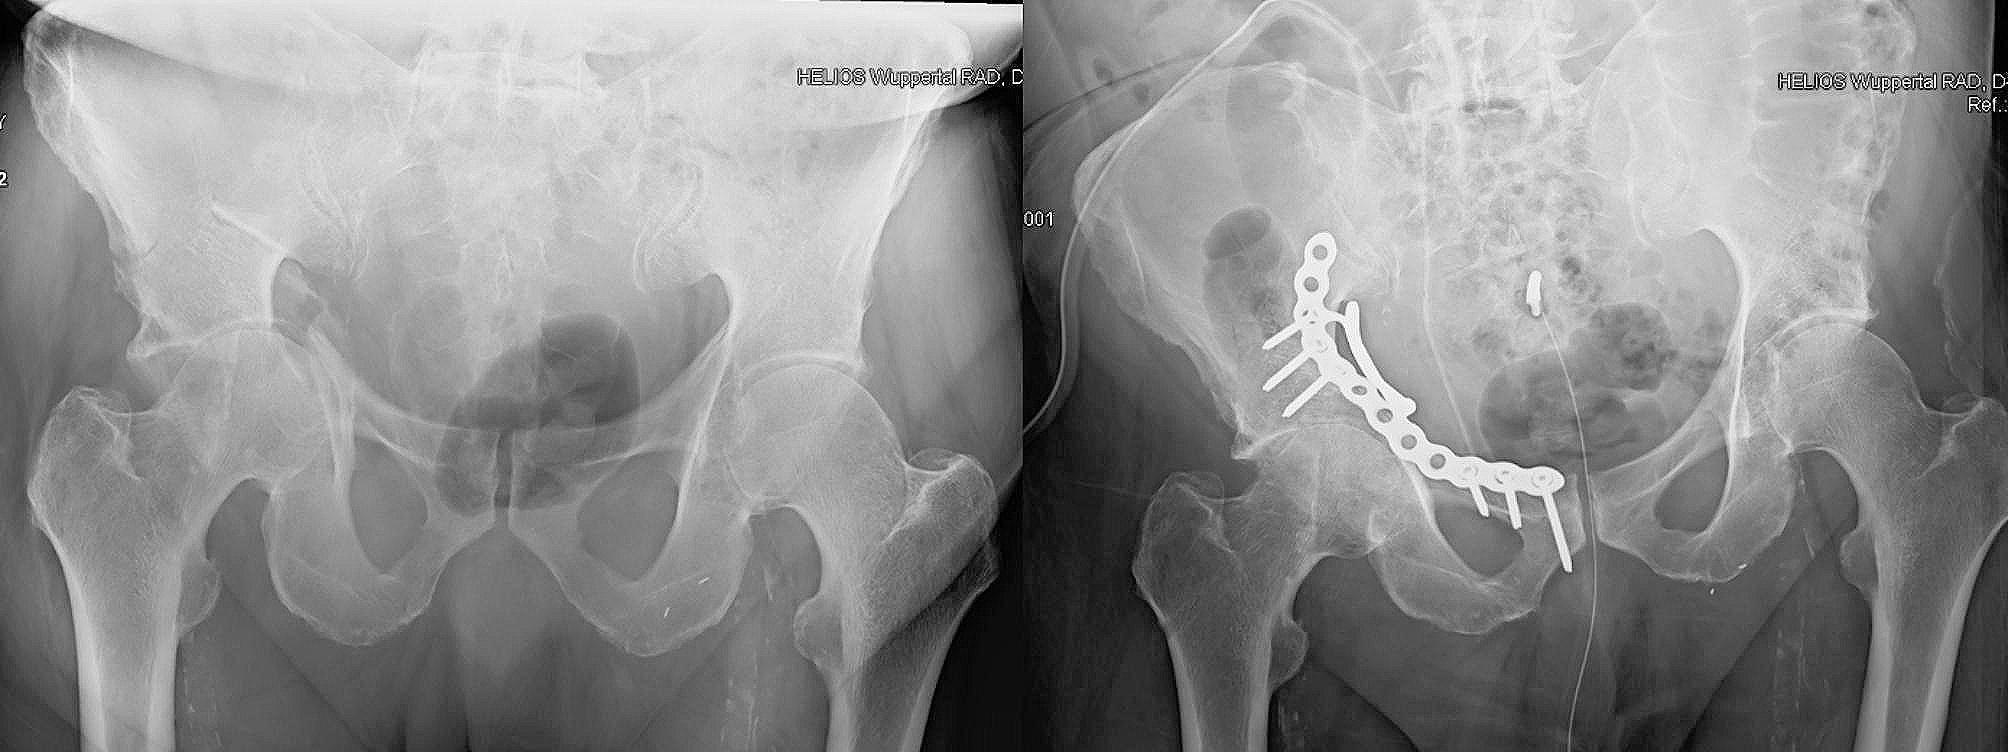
Preoperative X-ray image showing the fracture on the left and postoperative X-ray image showing the correctly positioned implant on the right
